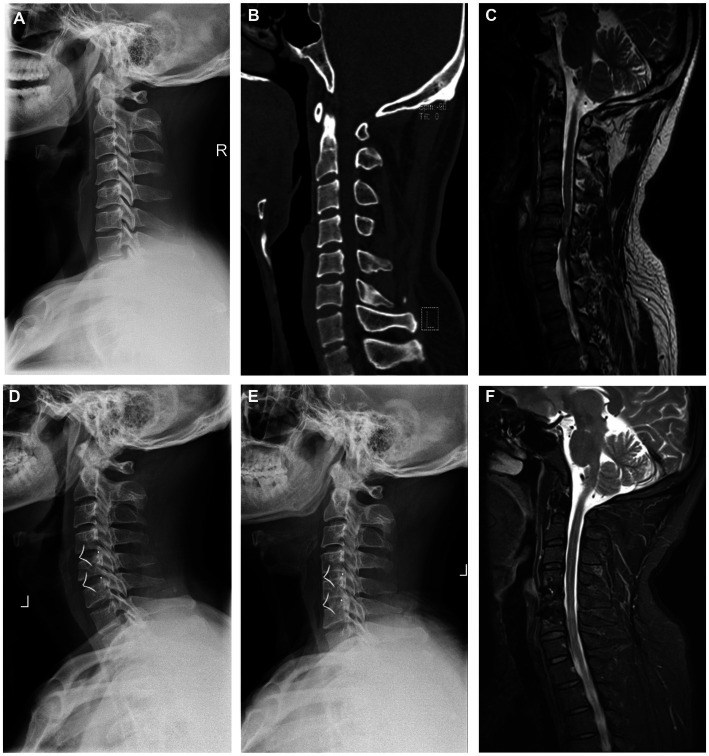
Images of a 49-year-old male patient at 88 months after anterior cervical zero-profile anchored spacer (ROI-C) surgery. Preoperative lateral cervical DR (A), CT (B), and MRI (C) indicate C4–C6 disc herniation with obviously spinal cord compressing. (D–F) Postoperative radiograph showed a good position of the internal fixation and corrected sagittal alignment at the final follow-up.
